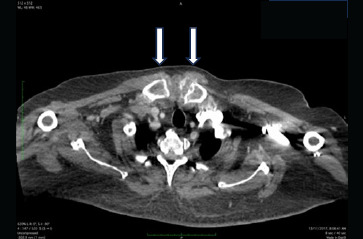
Axial view of the neck and thoracic CT scan showing bilateral soft tissue swelling at the head of the clavicles with calcification.
